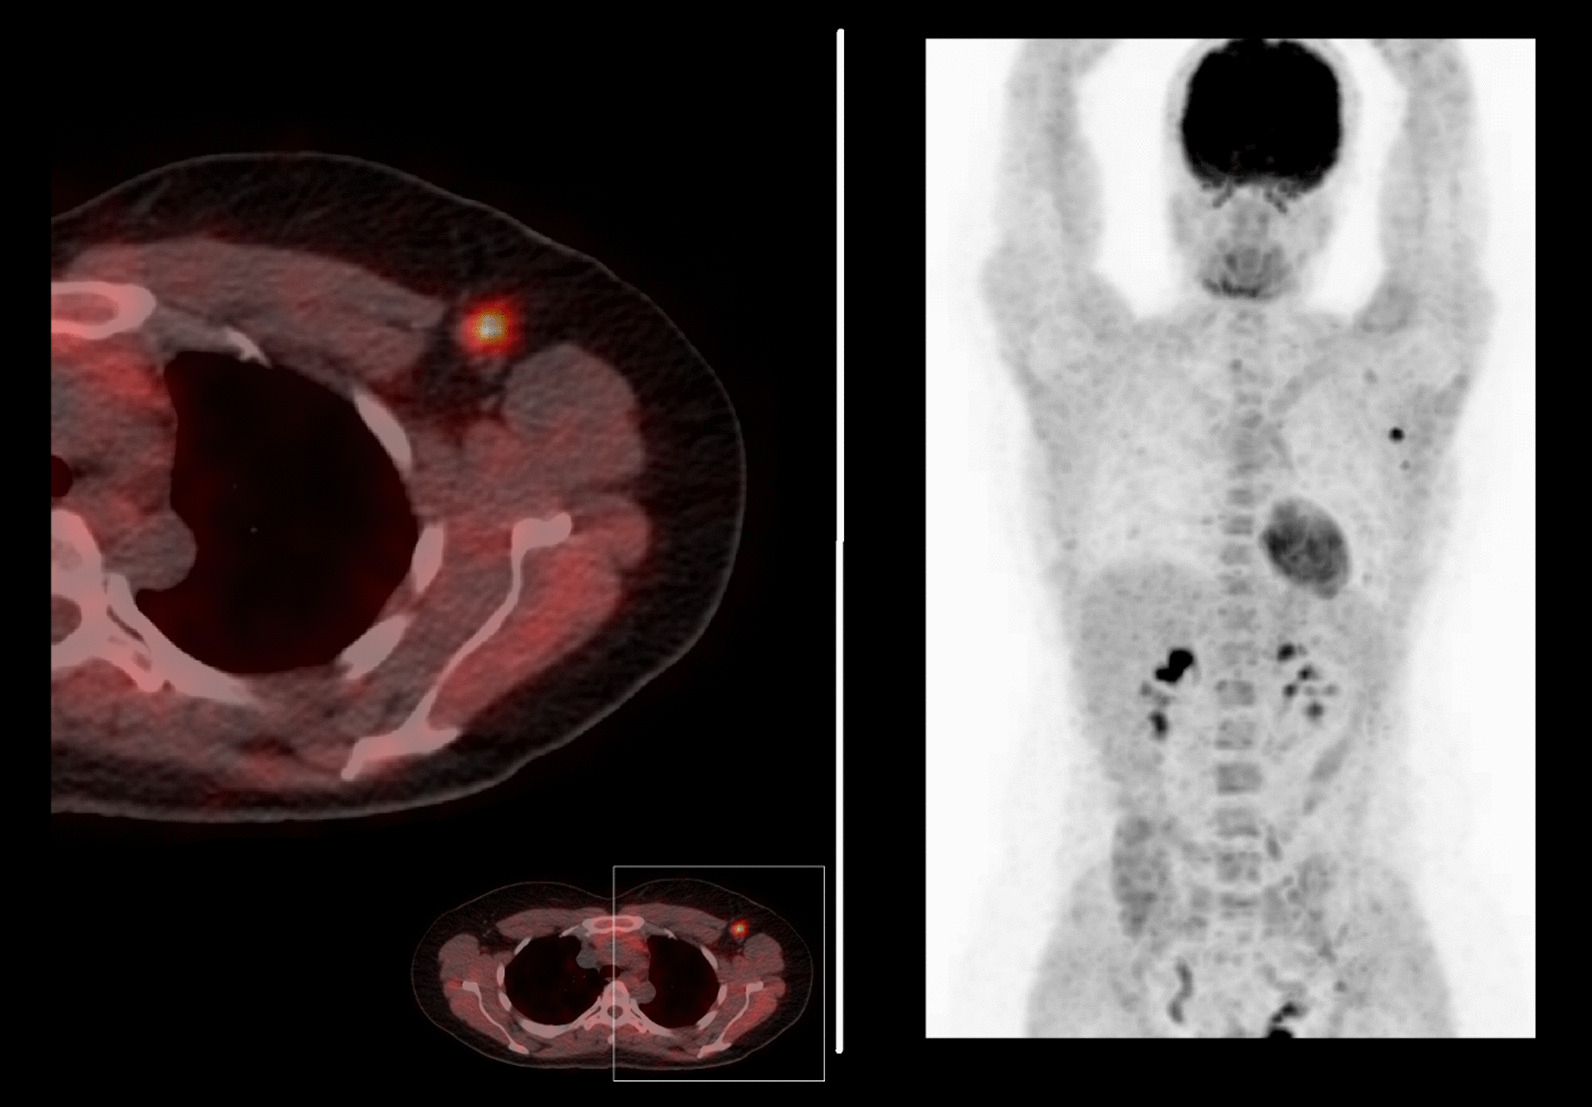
Patient example. Example of a patient with a 22-mm HER2-positive invasive carcinoma of no special type in her left breast. An axial ^18^F-FDG PET/CT exam of the left axilla shows the most FDG-avid axillary lymph node with an SUVmax of 7.37. Following completion NST, this patient had residual axillary disease
